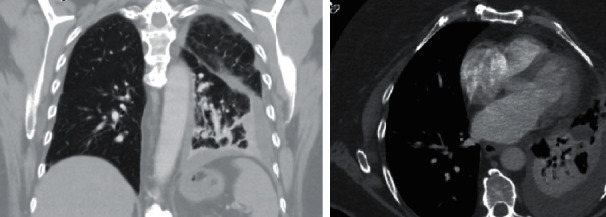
CT chest angiogram demonstrating extensive left lobar pneumonia with surrounding moderate-sized loculated pleural effusion.
